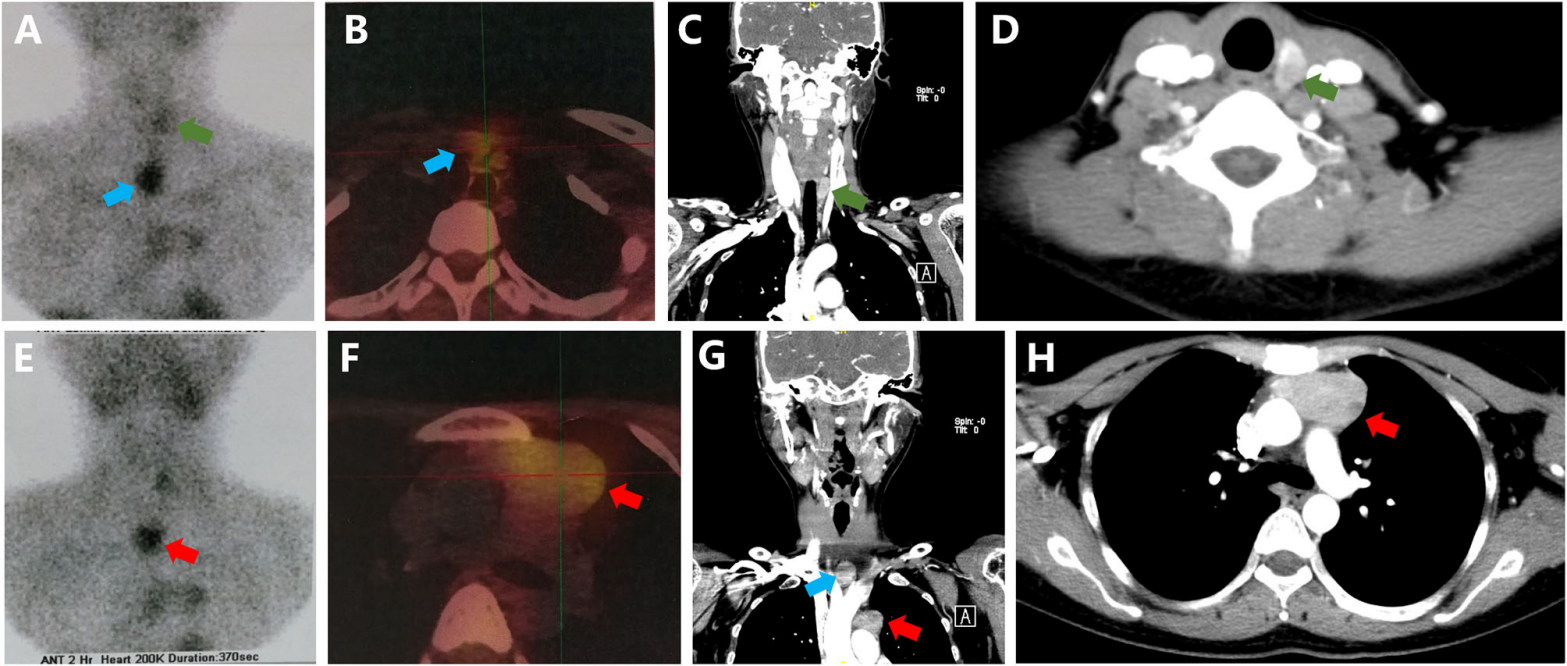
Imaging findings. (A,B,E,F) Technetium-99 methoxyisobutylisonitrile (99mTc-MIBI) imaging; (C,D,G,H) Enhanced computed tomography scanning. Green arrow for the parathyroid carcinoma in left inferior thyroid region, blue arrow for the carcinoma in the suprasternal fossa, and red arrow for the carcinoma in the thymic region.
